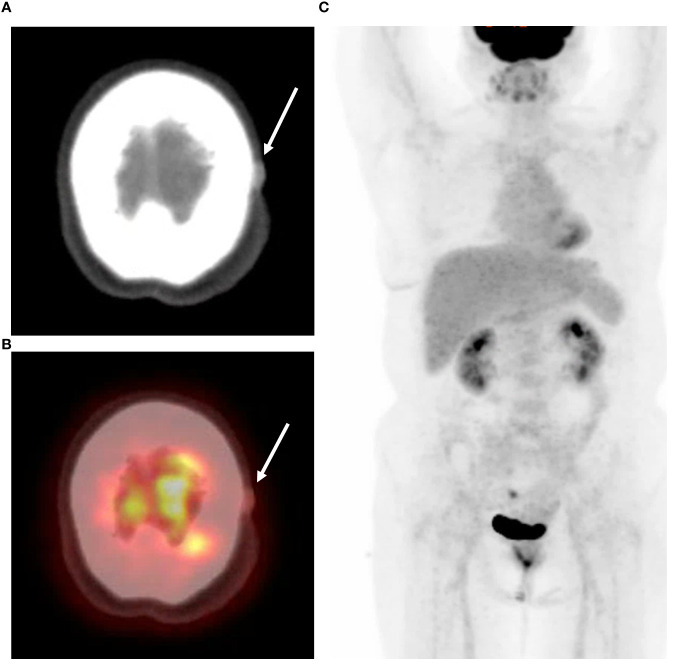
PET-CT findings of the patient. (A) A 1.5cm sized bulging lesion with soft tissue attenuation, involving subcutaneous layer of scalp along the left parietal area. (B) This lesion had a measured SUVmax value of 2, indicating a slight degree of hypermetabolism. (C) Maximum intensity projection images of PET-CT showed no evidence of other distant metastasis or recurrence.
